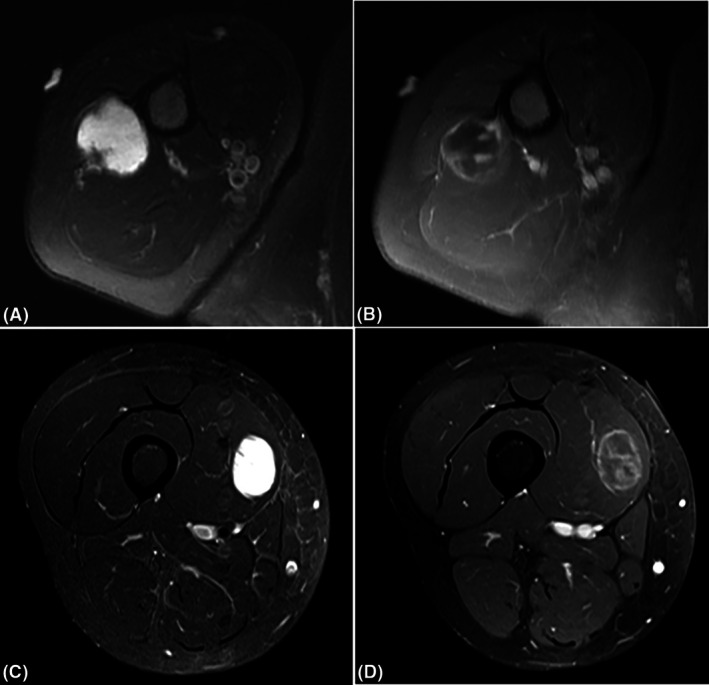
Representative radiographic images. Case 1: (A) Axial T2‐weighted fat‐suppressed image shows a well‐circumscribed, lobulated, extremely hyperintense intramuscular lesion involving the triceps. (B) Axial contrast‐enhanced T1‐weighted fat‐suppressed image demonstrates peripheral and septal enhancement. Case 2: (C) Axial T2‐weighted fat‐suppressed image shows a well‐circumscribed, extremely hyperintense intramuscular lesion involving the vastus medialis. (D) Axial contrast‐enhanced T1‐weighted fat‐suppressed image demonstrates peripheral and septal enhancement.
